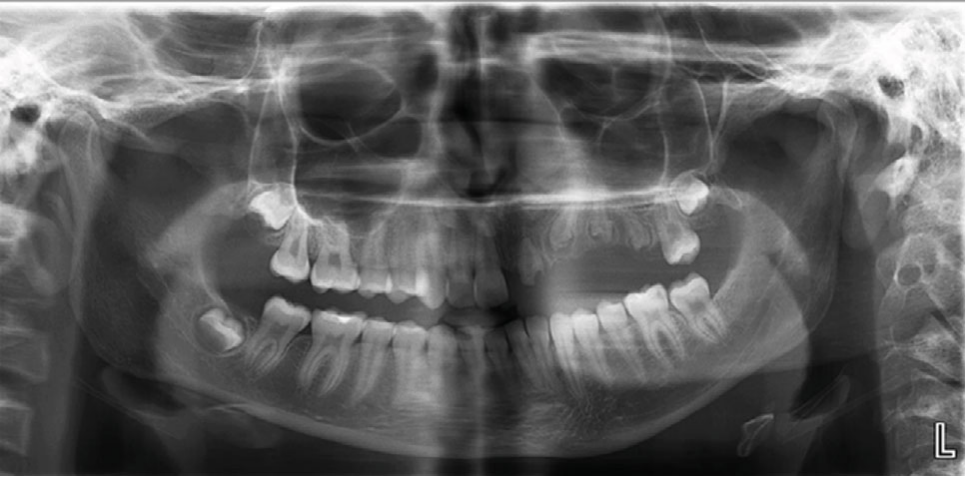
Orthopantomograph of the patient at age 15.
